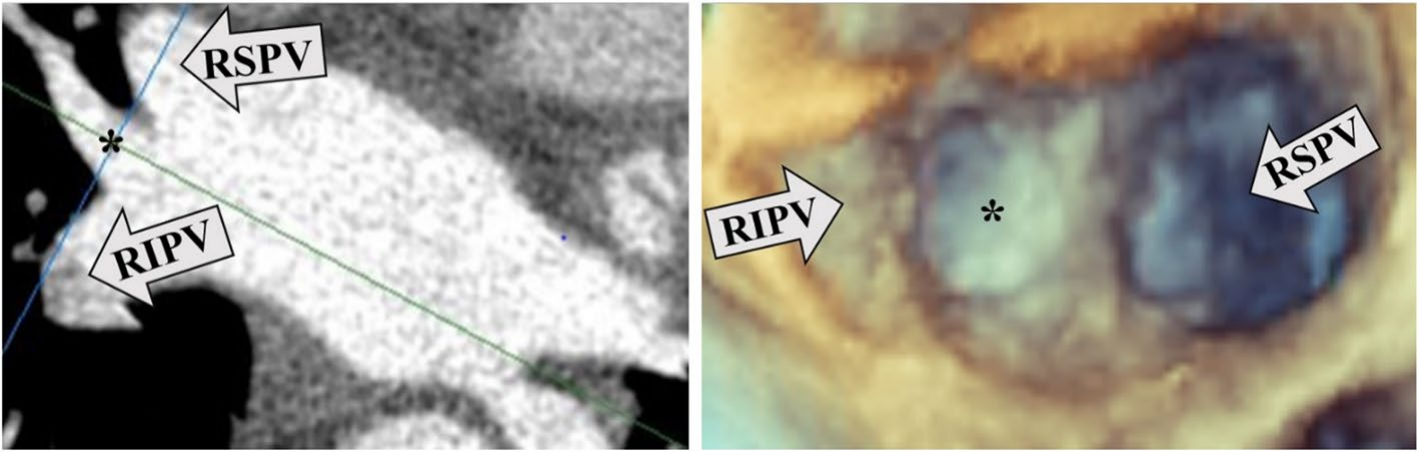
Supernumerary PV imaging. Supernumerary (right middle*) PV detected by CCT in 2D longitudinal plane (5a left) and by 3DTOE (full volume image, en face display; 5b right). *PV* (Pulmonary vein), *CCT* (Cardiac computed tomography), *2D* (Two-dimensional), *3DTOE* (Three-dimensional transesophageal echocardiography), *RSPV* (Right superior PV), *RIPV* (Right inferior PV)
